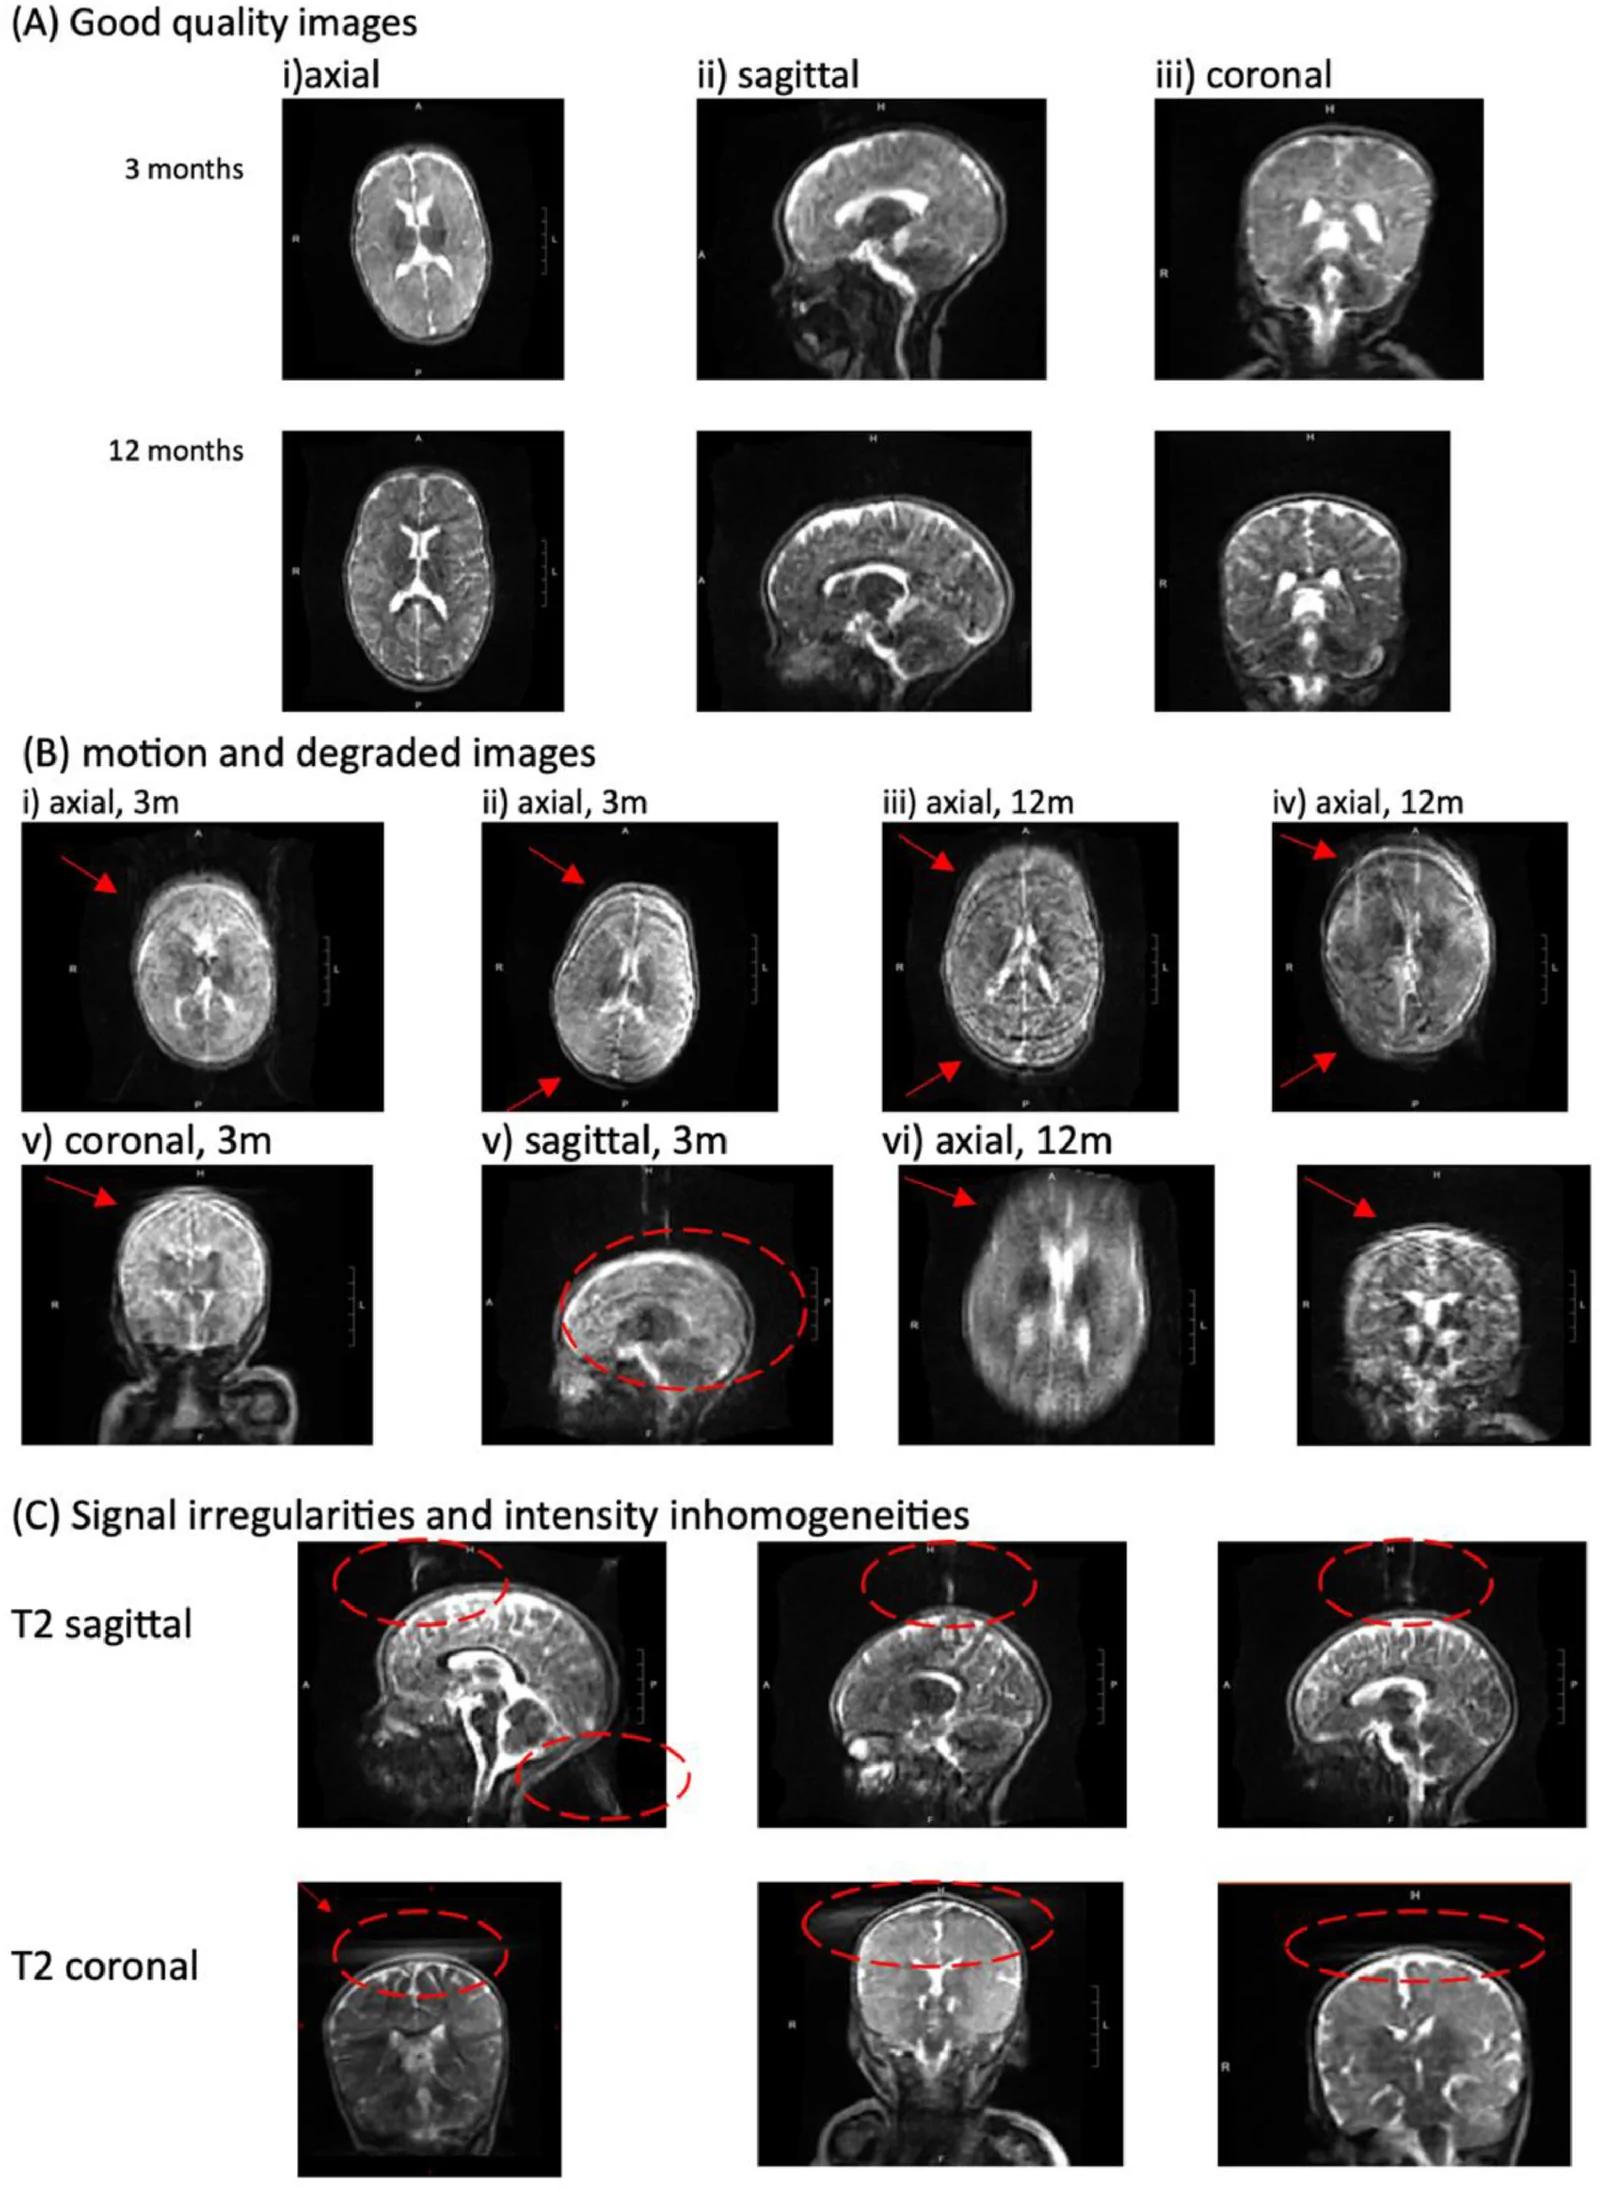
Representative image quality and artifact examples in infant ultra-low-field MRI.
